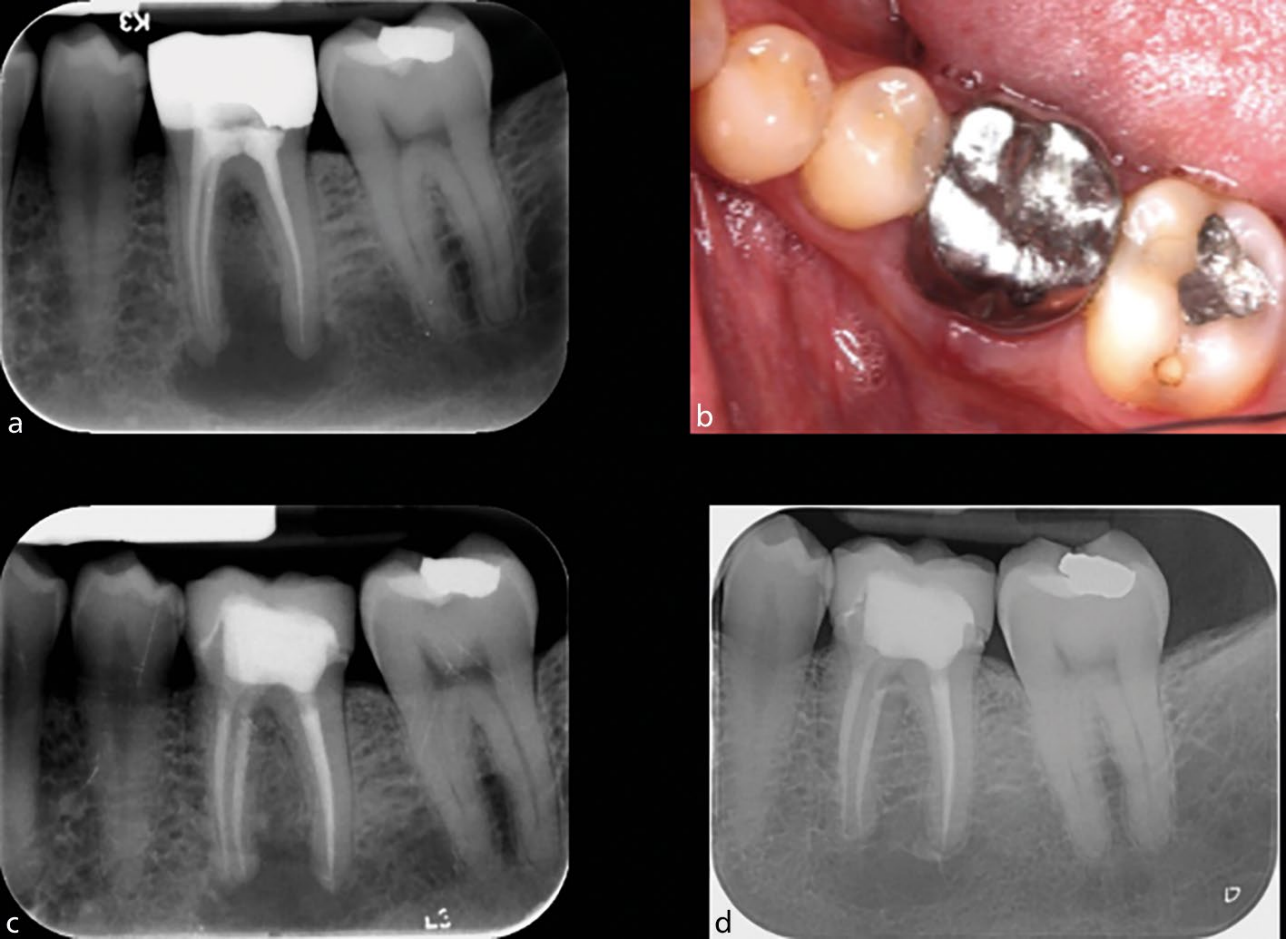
a, b, c) Root canal retreatment of this mandibular left first molar presenting with a large periapical lesion was carried out, after which a new crown was placed. d) At the ten-year review, clinical and radiographic examination reveals excellent healing. Image courtesy of Dr Kreena Patel
